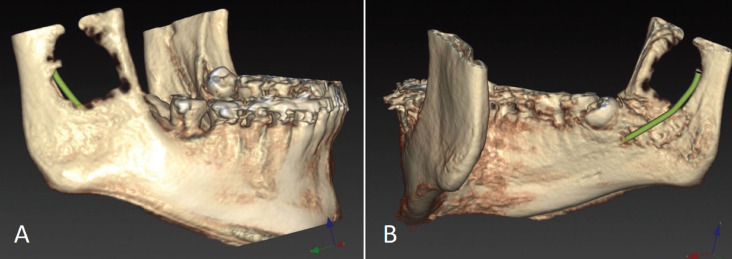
Cone-beam computed tomography of the simple bone cyst: 3D reconstruction. A) Buccal aspect, B) Lingual aspect.
